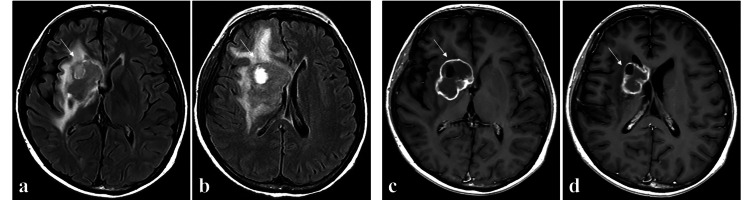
MRI acquired four months after surgery. Fluid-attenuated inversion recovery imaging (FLAIR)  (a, b) and gadolinium (Gd)-enhanced T1-weighted images (c, d) show shrinkage of the tumor (white arrow).
